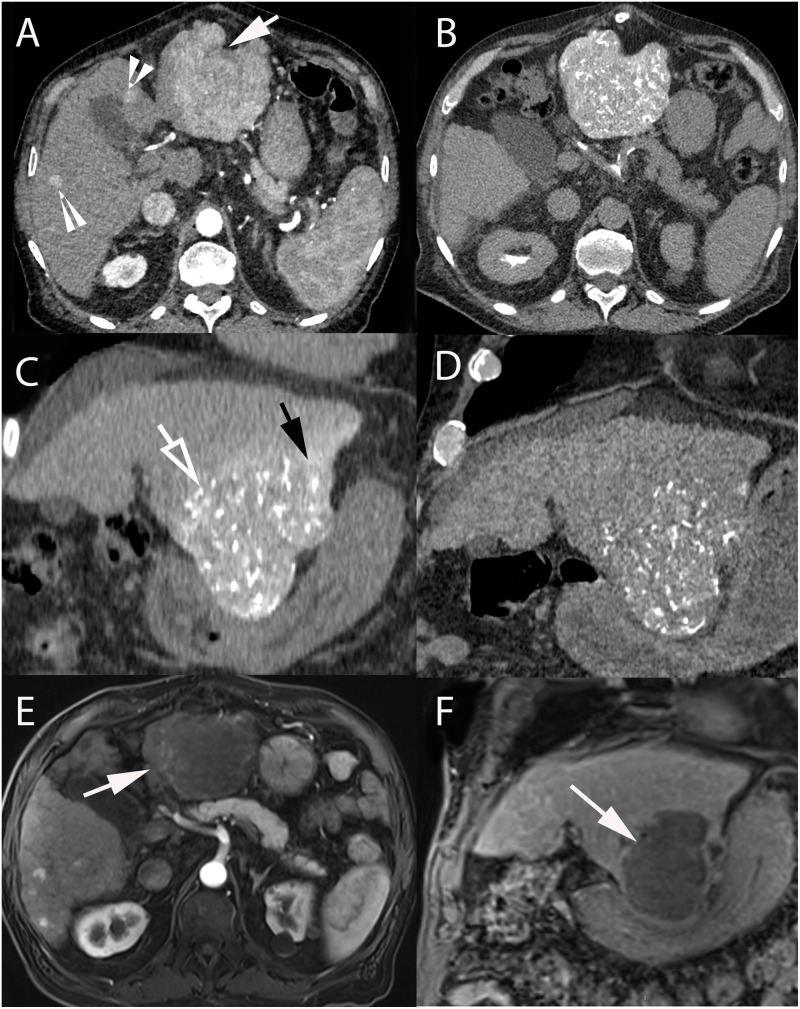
74-year-old male with hepatitis C cirrhosis and multifocal HCC treated with radiopaque drug-eluting beads loaded with doxorubicin. (A) Contrast-enhanced CT-scan (arterial phase) showing a large HCC in segments II-III (arrow) and small HCC lesions in segments IV and V (arrowheads). Axial (B) and coronal (C) unenhanced CT after selective administration in the left hepatic artery clearly demonstrating two types of attenuation: from radio-opaque drug eluting-beads (DC Bead LUMI loaded with doxorubicin) deposited into the tumor (hollow arrow) and soluble contrast medium used during catheterization and embolization (black arrow). (D) Coronal unenhanced CT image at 1-month post TACE showing that radiopaque beads deposition was still readily visible while the soluble contrast medium had long “washed out.” Axial (arterial phase) (E) and coronal (portal phase) (F) contrast-enhanced T1-weighted MRI performed at 1-month after 2 consecutive TACEs demonstrated extensive necrosis of the treated lesion (white arrow).
